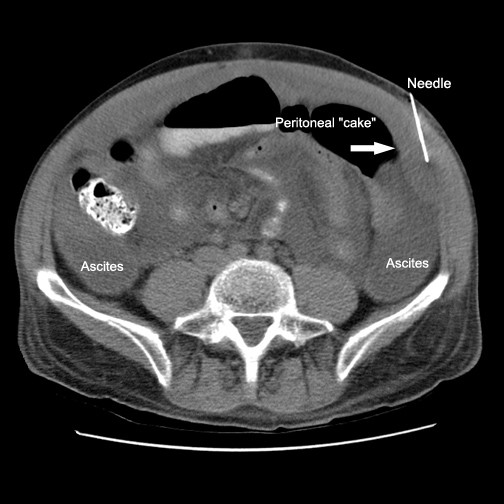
CT image showing loculated ascites, FNA needle and omental/peritoneal "cake".
